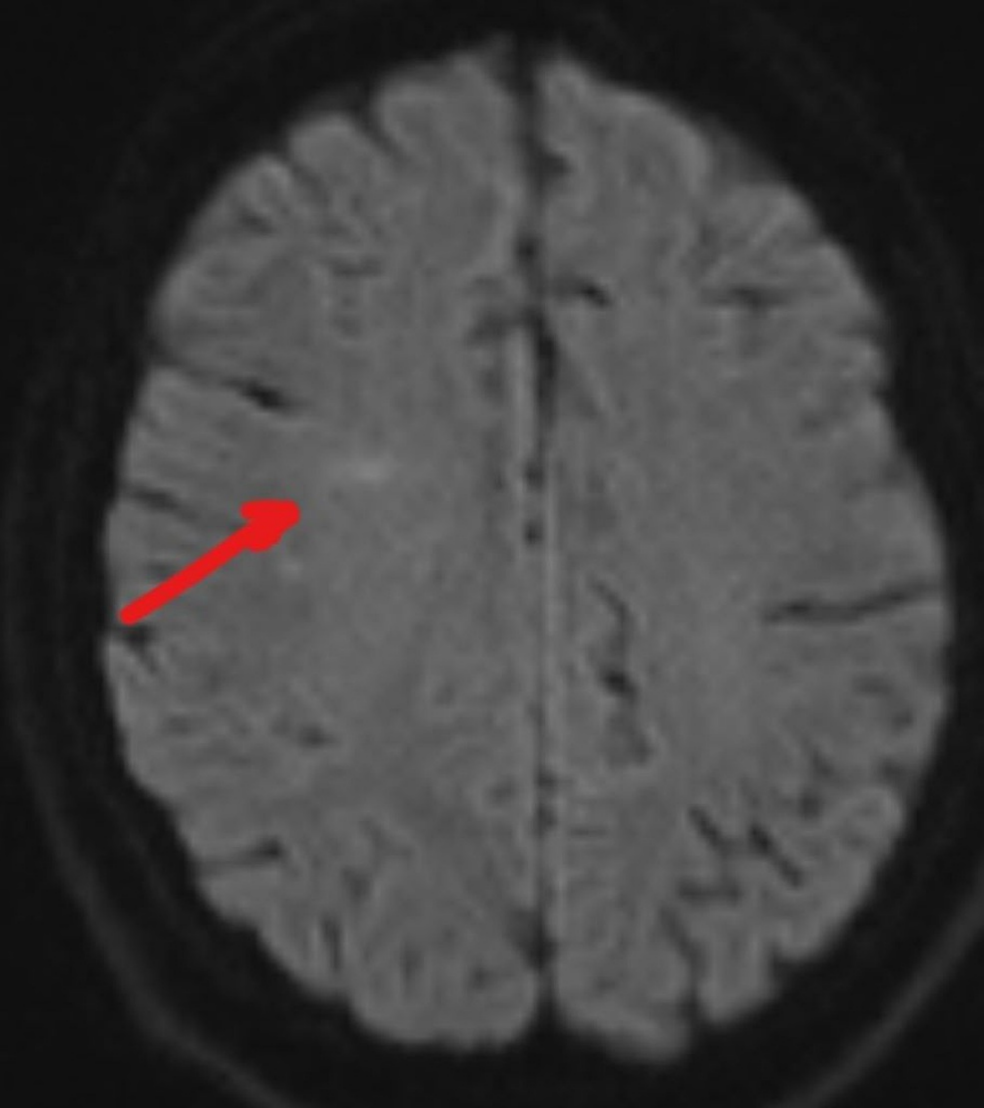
DWI of head MRI, Day 4. DWI: diffusion-weighted imaging.
